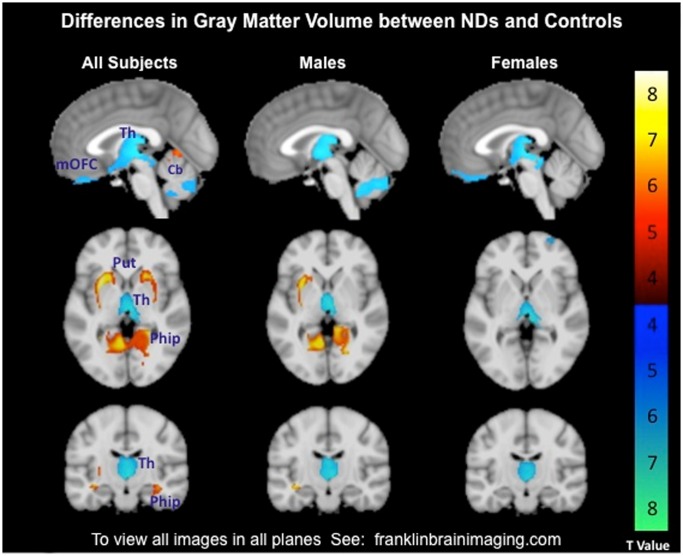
Differences in gray matter volume (GMV) between nicotine dependent (NDs; n = 80) and control subjects (Controls; n = 80) shown in all subjects and separately by sex (NDs, n = 41 male; Controls, n = 41 male). Representative MRI sagittal, axial, and coronal brain slices analyzed in SPM8 and overlain on the Montreal Neurological Institute brain. Slice shown is centered within the thalamus (x, y, z; 0, −16, 0). Hot colors represent regions of greater GMV, while cool colors represent regions of less GMV in NDs compared to Controls. T values range from 4.42 to 8.06, significant in whole brain analysis at *p*<0.001, and FWE cluster-corrected *p*<0.025. Images are displayed neurologically. An interactive visual display of all brain data in all three planes can be found at http://franklinbrainimaging.com (Available upon publication).
